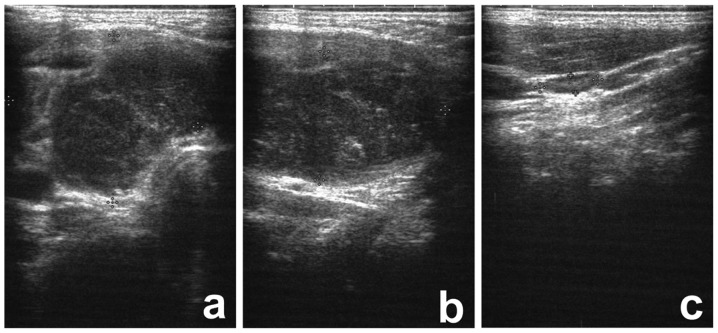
B-ultrasound scan. A mass was detected in the thyroid tissue with (A) the short axis measuring 31.2×26.2 mm and (B) the long axis measuring 39.9×20.3 mm. (C) The surrounding lymph nodes were normal and the largest measured 9.6×2.9 mm in size.
